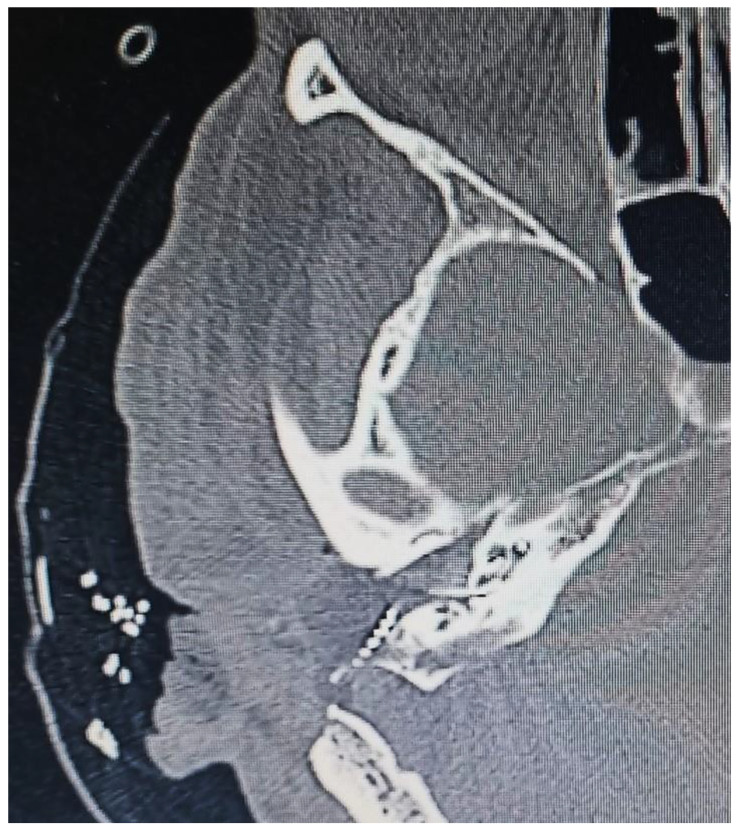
Postoperative Axial Head CT revealing correct electrode array insertion in the right cochlea.
